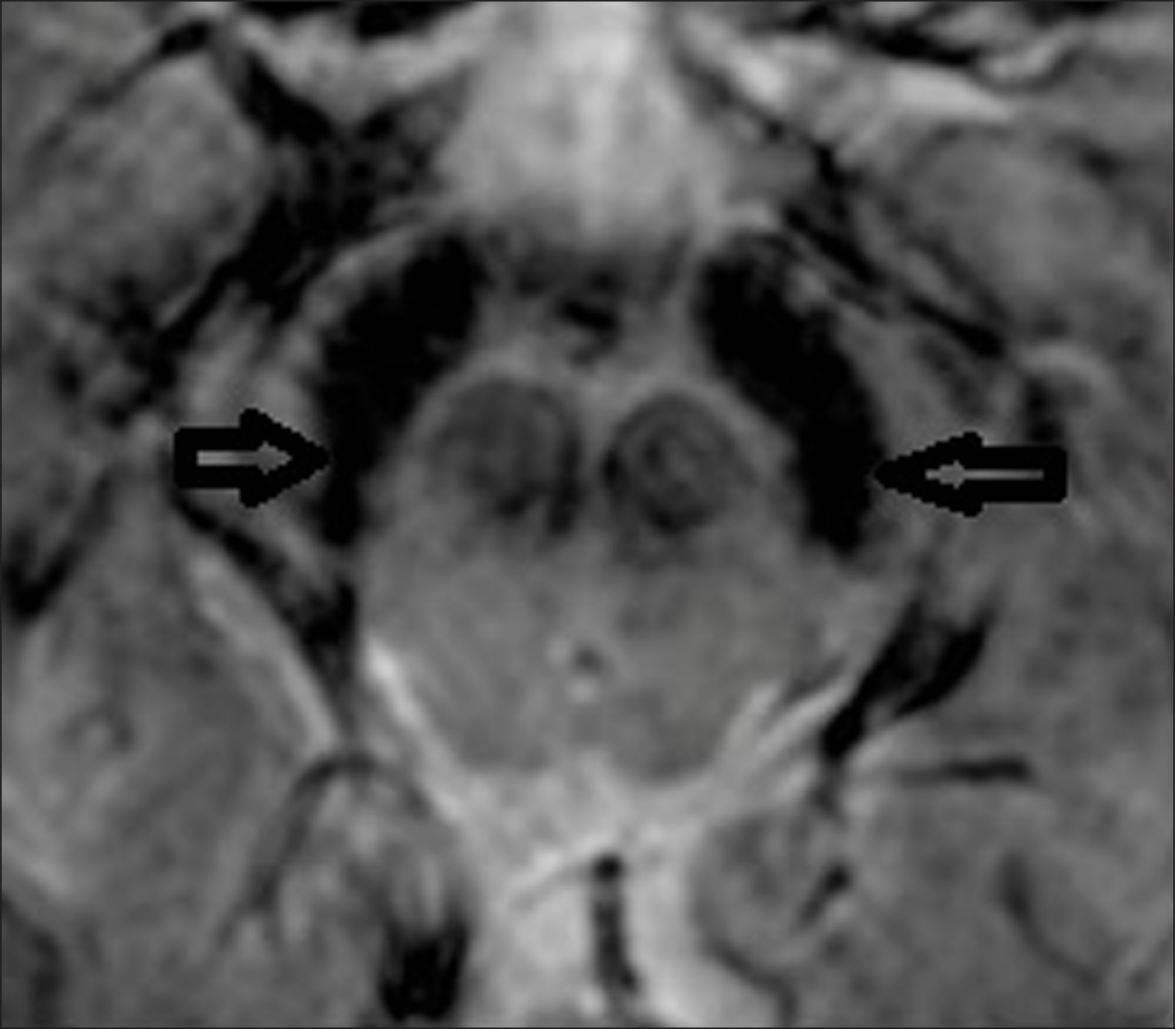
Axial Mecho 3T MRI, 51-year-old male with Parkinson Disease and 5 years of disease. Bilateral Absence of N1 (arrows).
